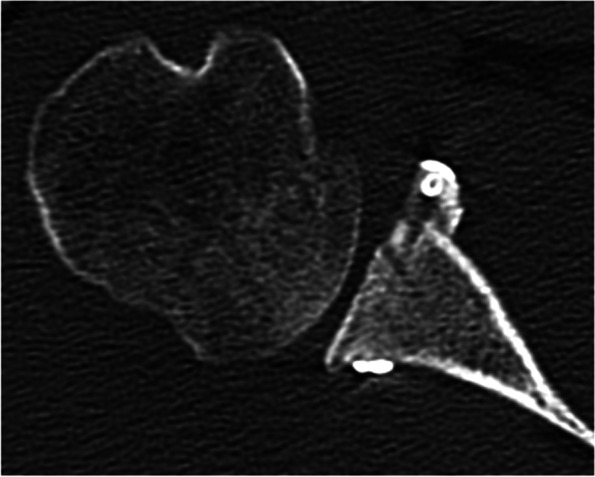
Postoperative CT-scan of a patient with an off-track Hill-Sachs lesions who underwent an arthroscopic Eden-Hybinette procedure. Courtesy of Dr Miguel García Navlet, Hospital Asepeyo Coslada (Madrid)
